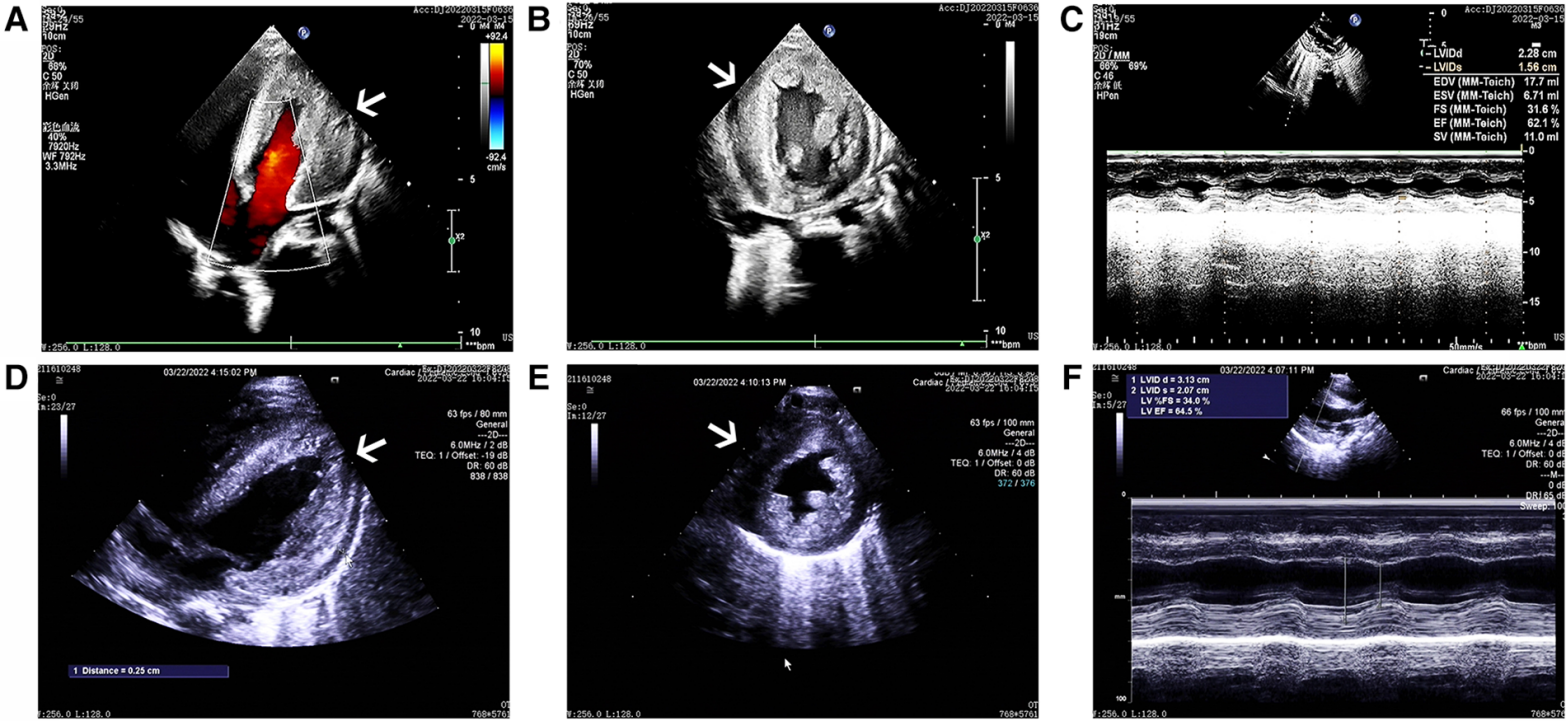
The ultrasound echocardiograms of the patient showed significant myocardial hypertrophy. The images (A–C) sequentially represent the apical four-chamber view, the left ventricular short-axis view, and the M-mode ultrasound changes before treatment. Similarly, images (D–F) represent the same views and changes after treatment.
